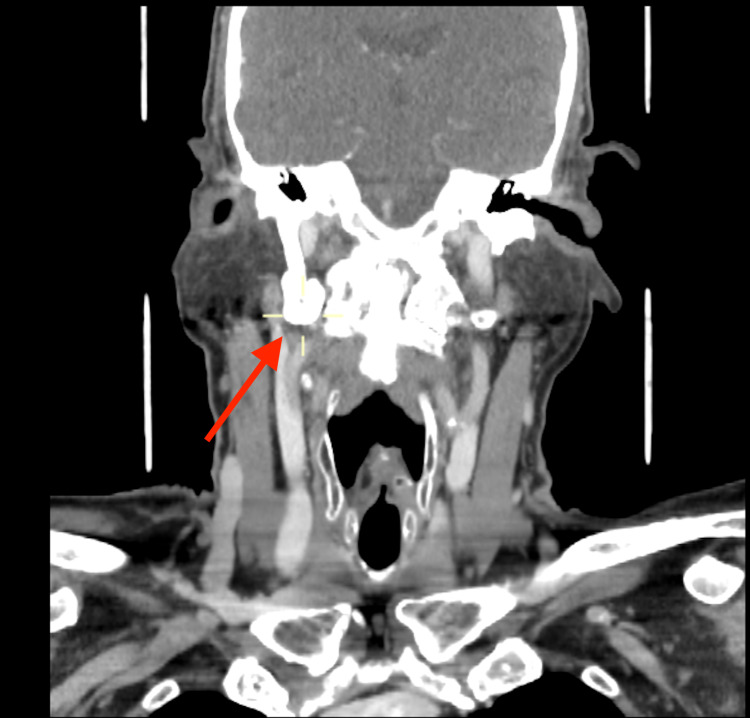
Follow-up CT of the neck and thorax with contrast (coronal plane), showing complete ossification of the stylohyoid ligament and significant bony overgrowth (red arrow).
